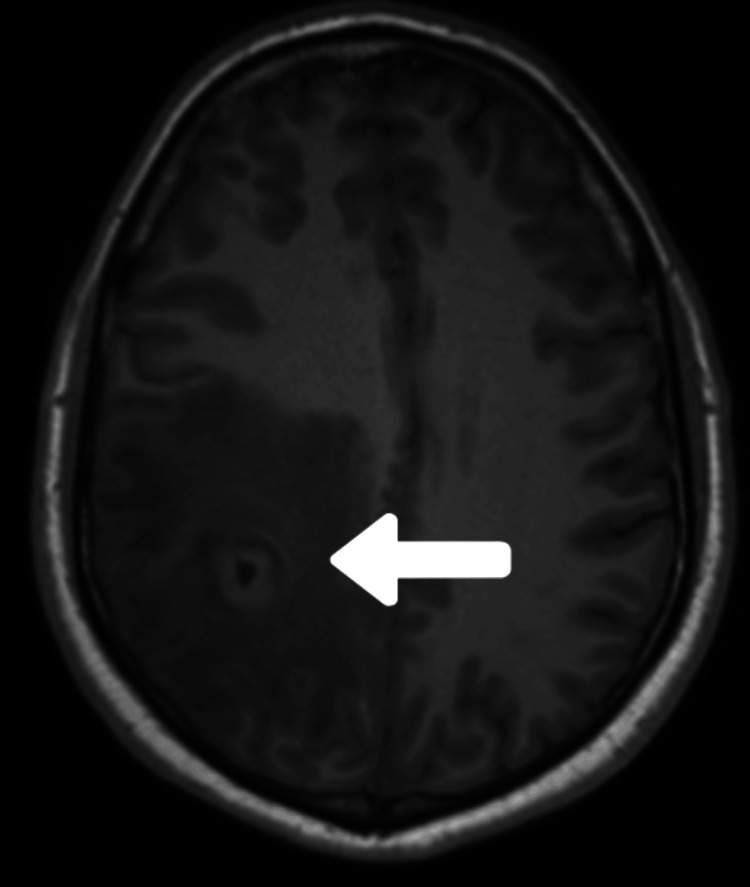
T1-weighted MRI demonstrating vesicular cysticercus Axial T1-weighted MRI demonstrates a single vesicular stage of cysticercus (white arrow) in the right frontoparietal lobe. MRI: magnetic resonance imaging
